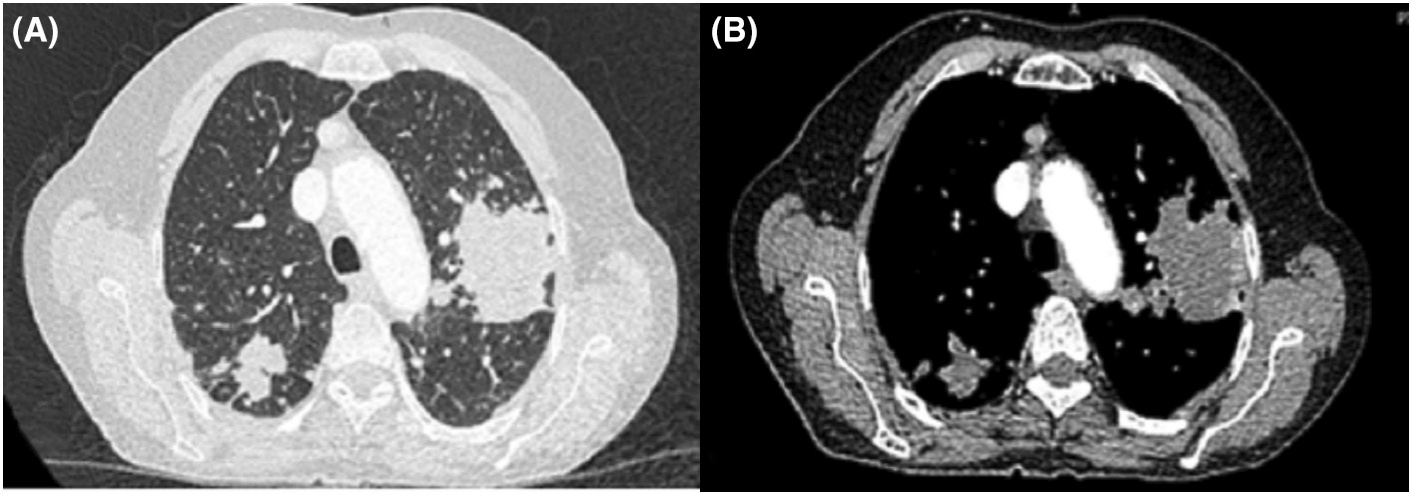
(A) Chest CT, axial cut, lung window: multifocal patchy consolidation with surrounding nodular opacity in both lungs. The largest is in the left lung in the upper lobe which shows relatively speculated outlines. No significantair bronchogram is noted. Centriacinar emphysematous changes of the lungs are seen. (B) Contrast enhanced chest CT, axial cut, mediastinal window: multifocal patchy non‐enhancing consolidation with surrounding nodular opacity. No significant air bronchogram is noted. Irregular speculated outlines of the opacities are seen.
